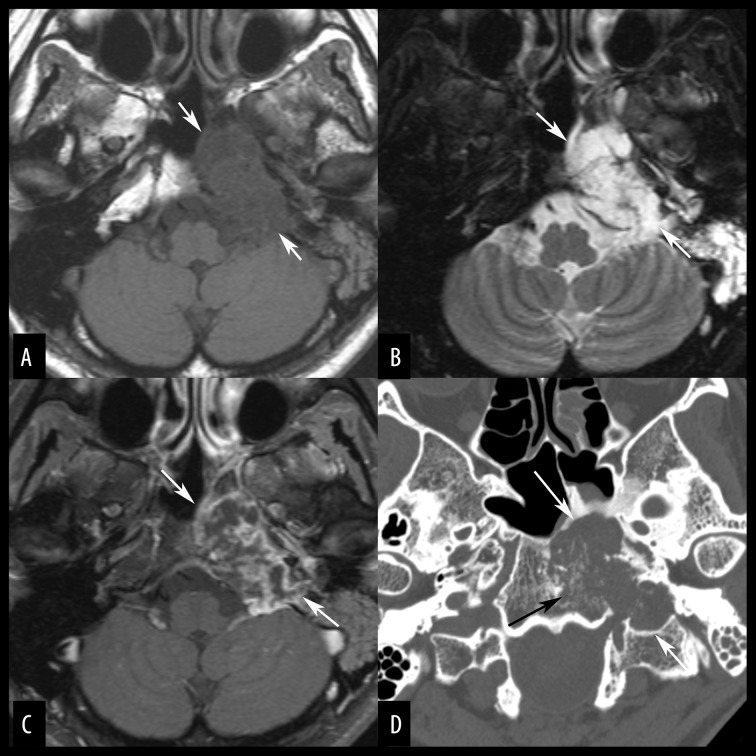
Skull base chondrosarcoma in a 46-year-old woman. (A) Axial, non-contrast, T1-weighted MR image shows an expansile, hypointense mass (arrows) engulfing the left petrous apex and the clivus. The epicenter of the tumor is likely located at the left petroclival fissure. (B) On an axial, fat-suppressed, T2-weighted MR image, most of the tumor shows very high signals (arrows). The left mastoid air cells lose aeration, likely because the Eustachian tube is obstructed. (C) Axial, contrast-enhanced, MR image with fat suppression shows peripheral enhancement (arrows). (D) On an axial, bone window CT image, punctuate calcification is seen in the tumor (black arrow). The margin of the tumor is rather distinct (white arrows).
